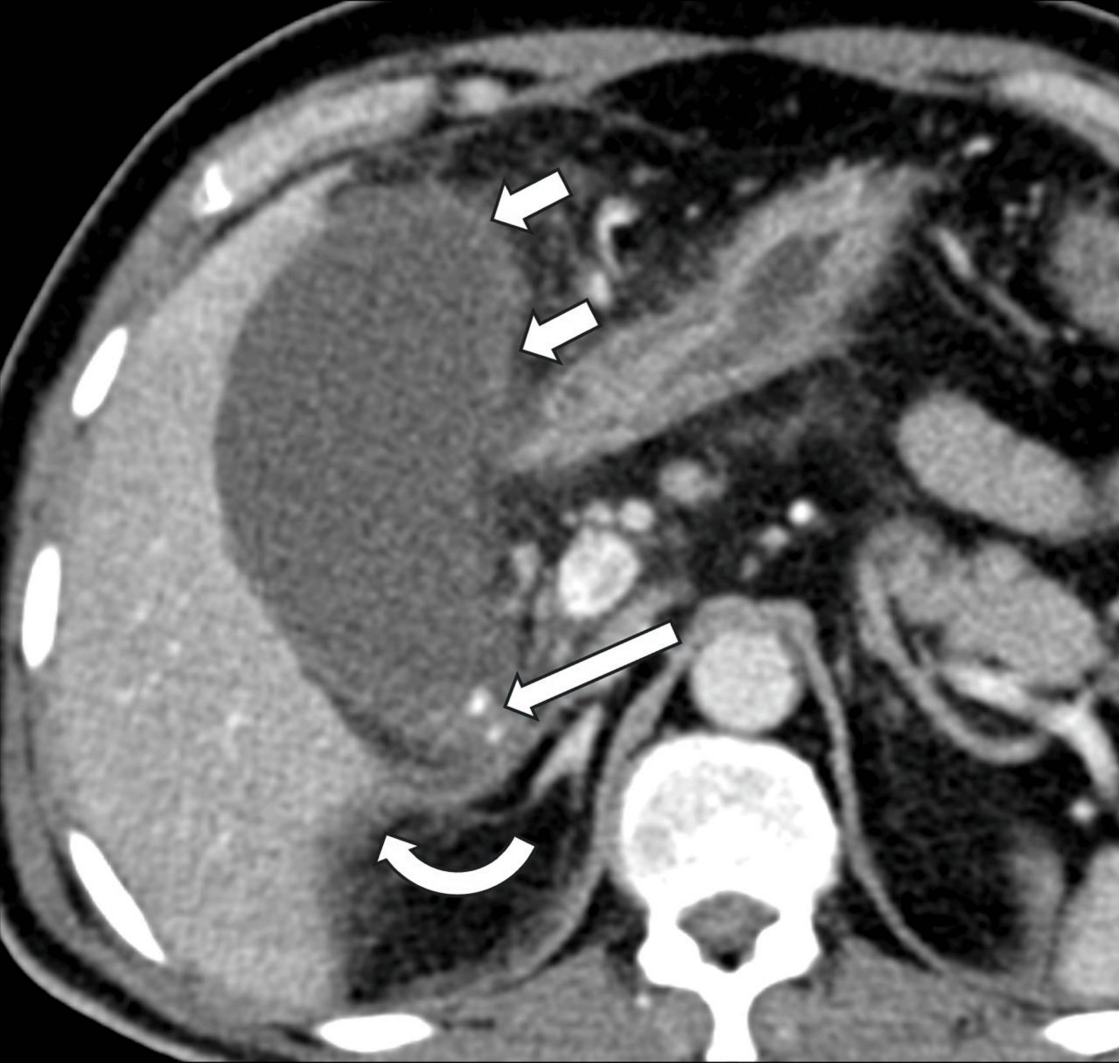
Contrast-enhanced abdominal CT scan (axial image) Contrast-enhanced abdominal CT scan (axial image) one day after ultrasound showing an over-distended gallbladder with sludge and stones (long arrow) at the gallbladder neck. The gallbladder wall is non-enhancing and irregular (short arrows), with probable micro-perforation. There was no mural or luminal gas. Adjacent fat stranding and traces of free fluid are also noted (curved arrow).  The appearance is consistent with gangrenous cholecystitis.
